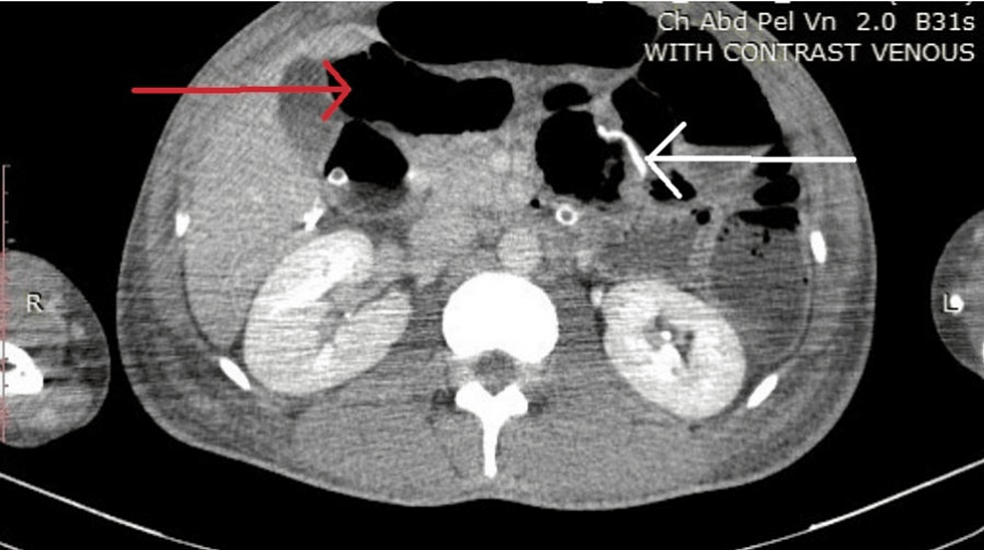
Axial slice of the computed tomography scan with intravenous contrast in the venous phase showing dilated loops of the small bowel and the suture line of the ileocolic anastomosis White arrow - ileocolic anastomotic suture line; red arrow - dilated loop of small bowel
